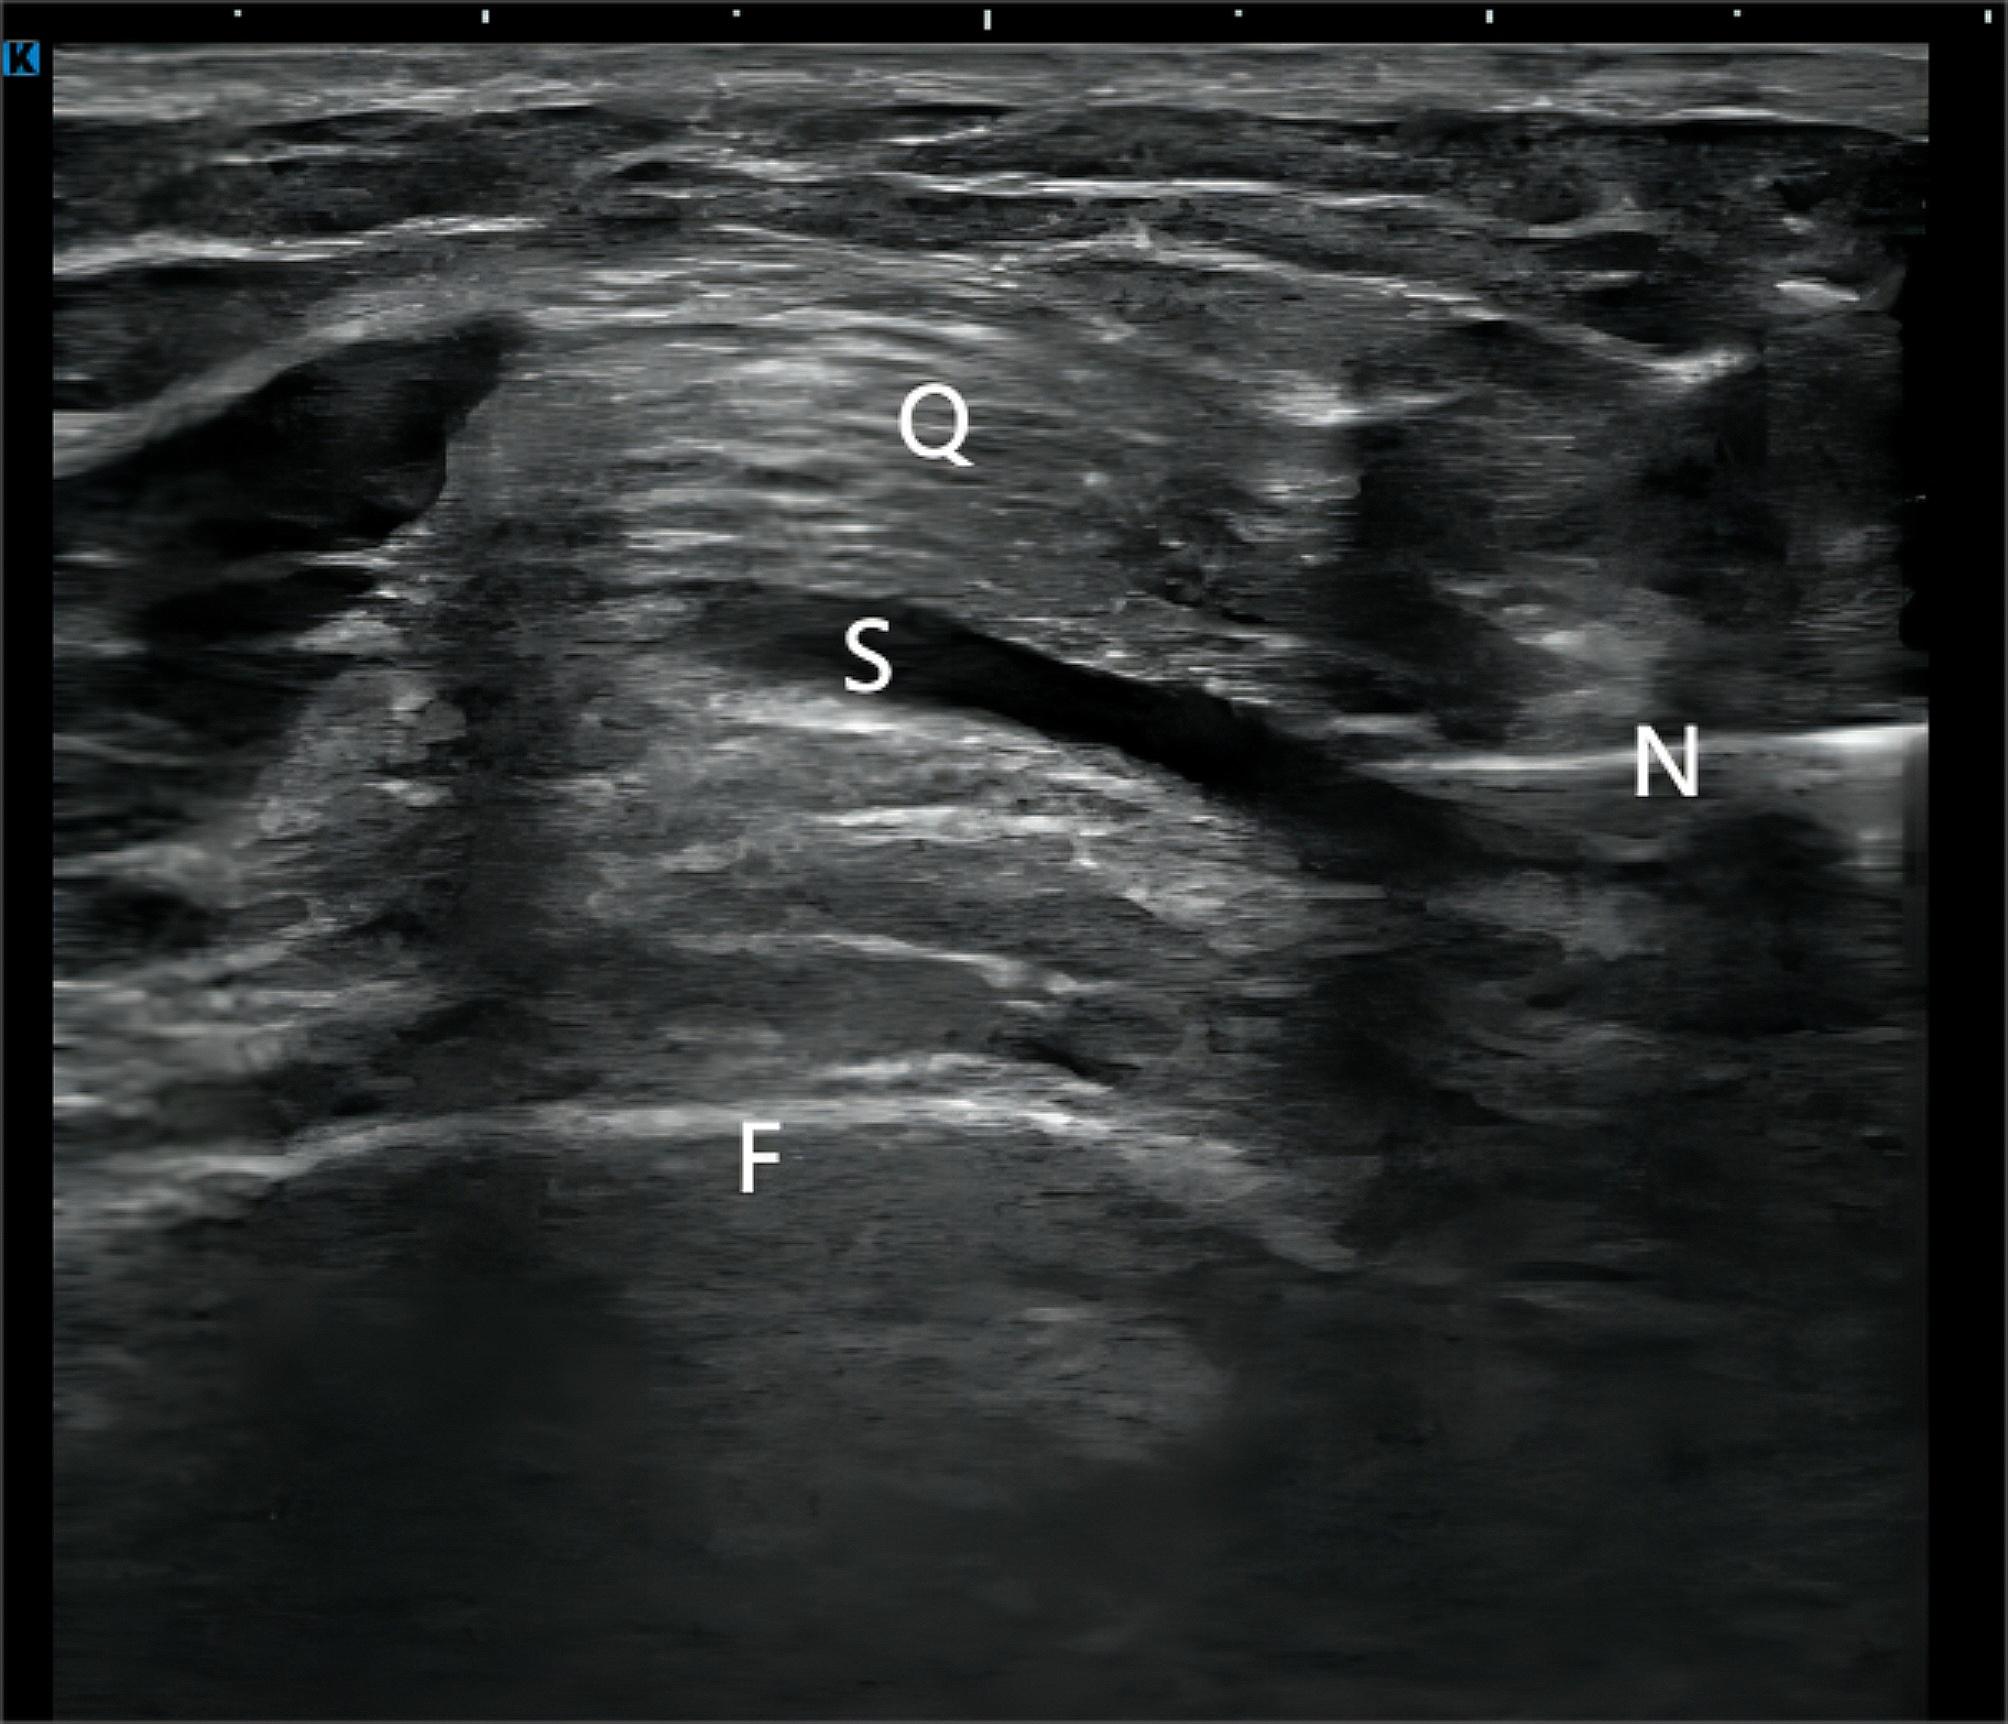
Platelet-rich plasma injection guided by ultrasound. F: Femur, Q:Quadriceps tendon, N:Needle, S:Suprapatellar bursa
